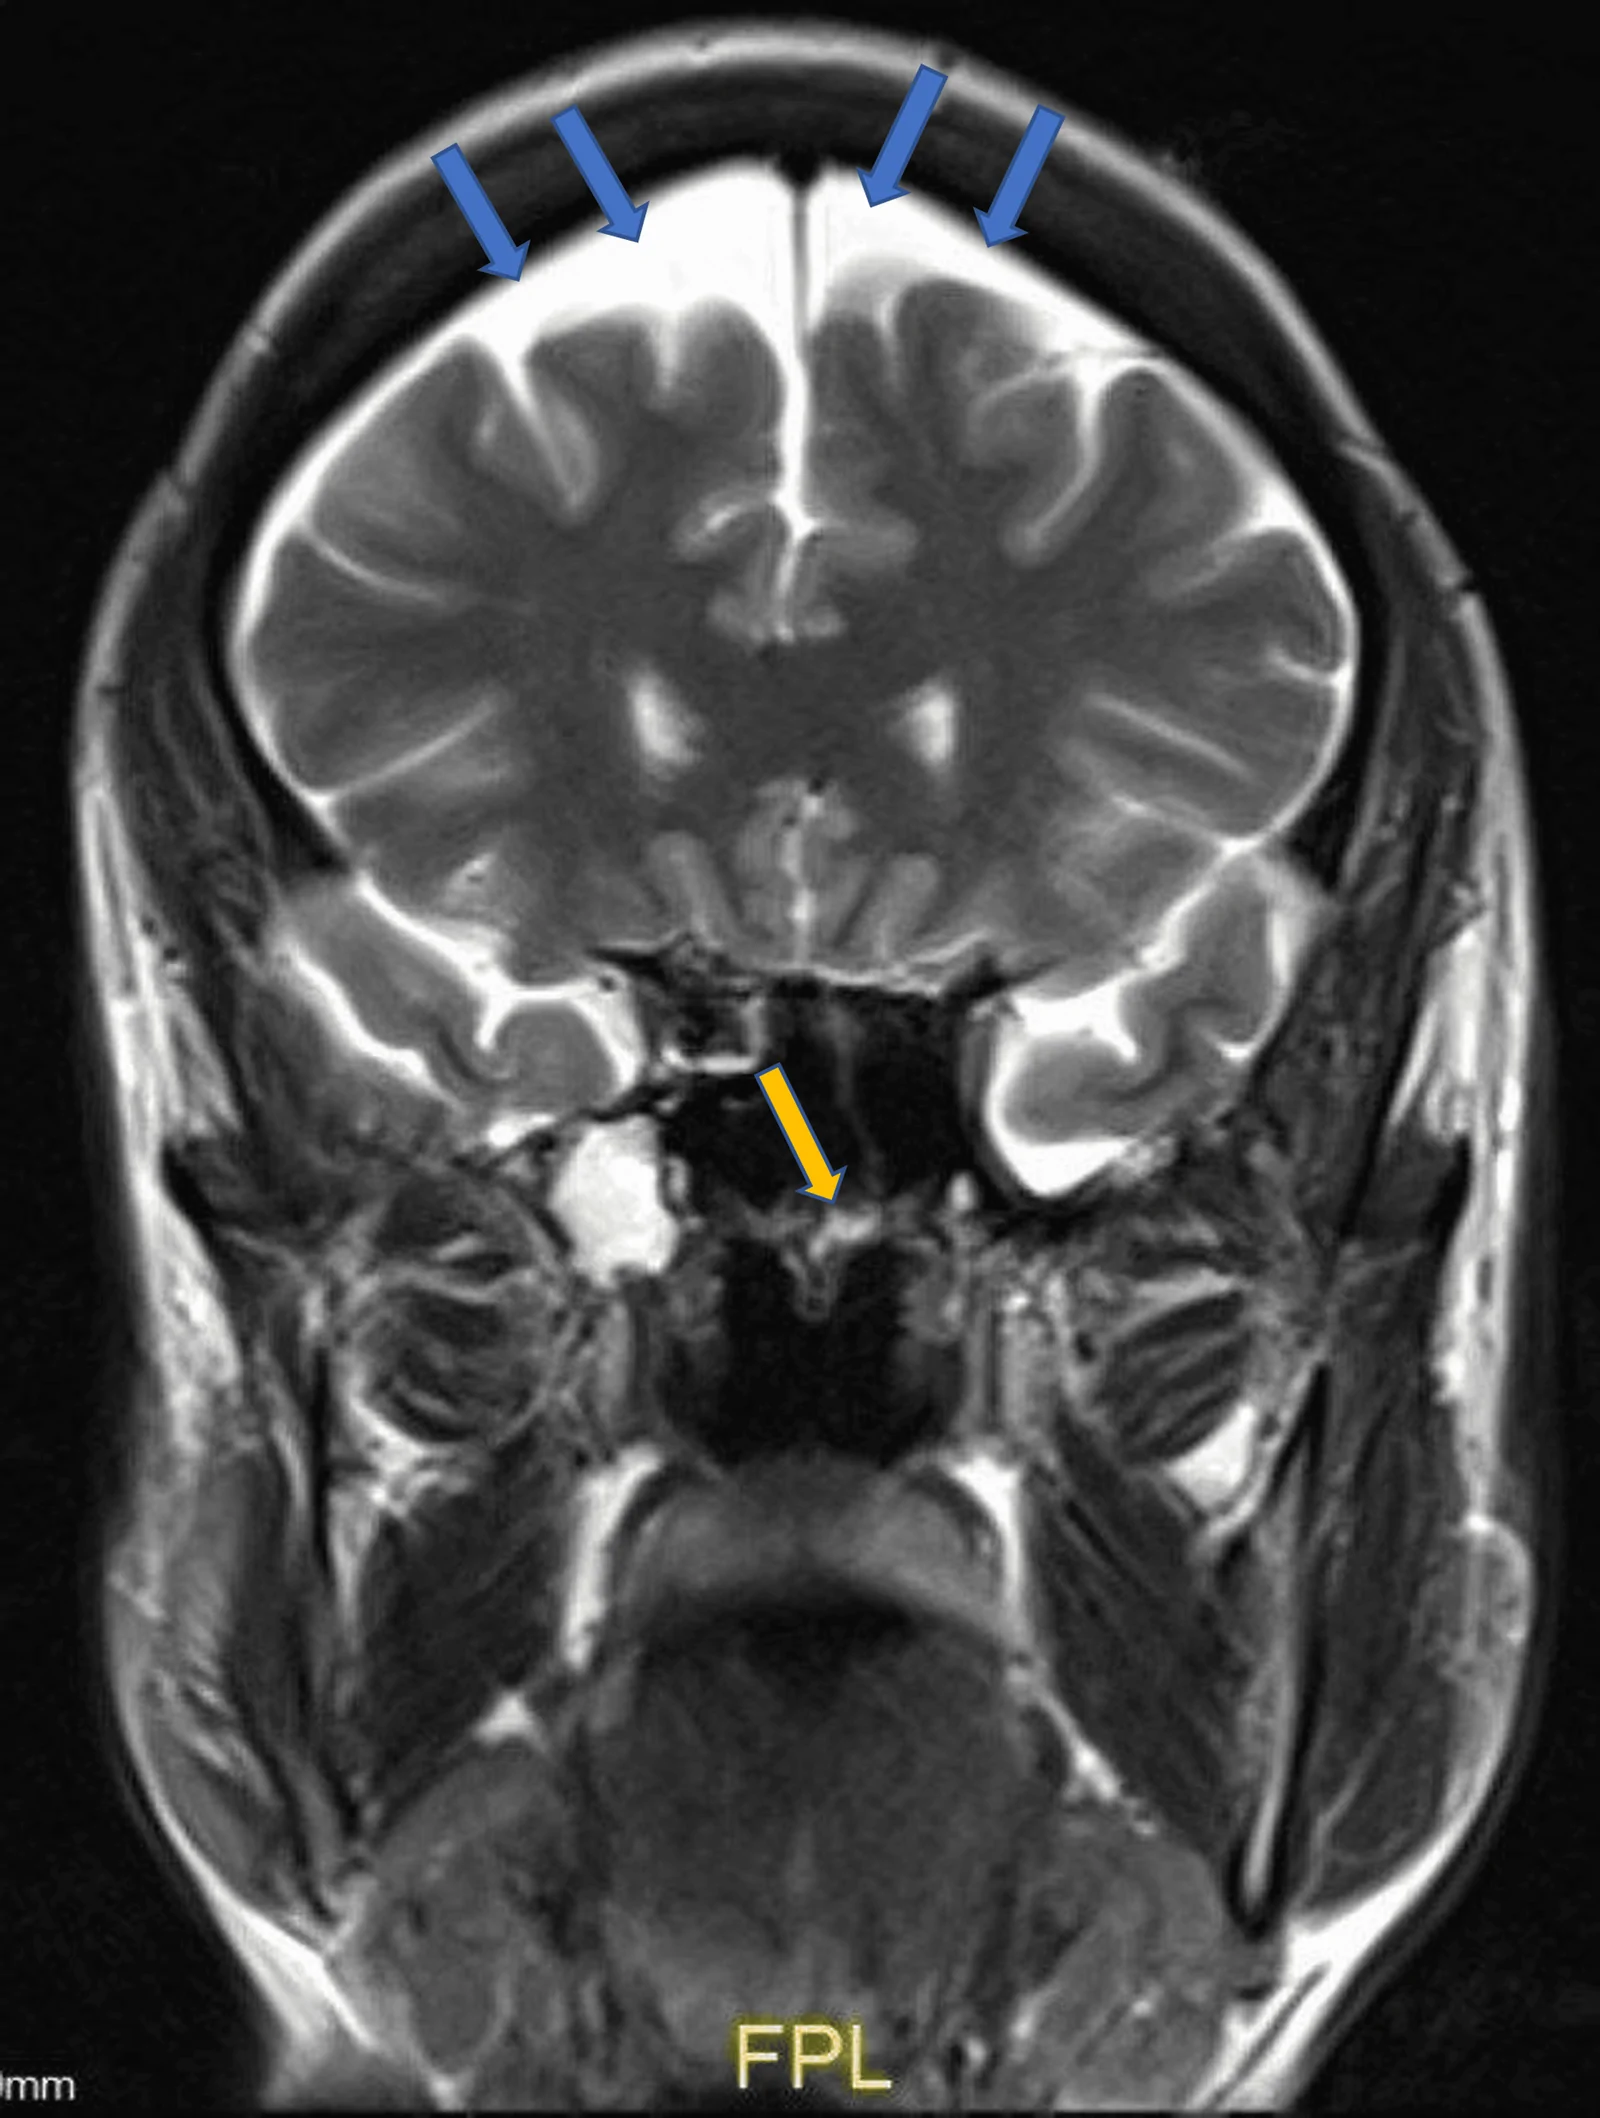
Follow-up MRI brain without contrast, coronal T2 (two months). Blue arrows: expanded frontoparietal extra-axial CSF spaces. Orange arrow: partial empty sella. Findings stationary compared with the acute admission MRI, confirming a pre-existing chronic condition.
